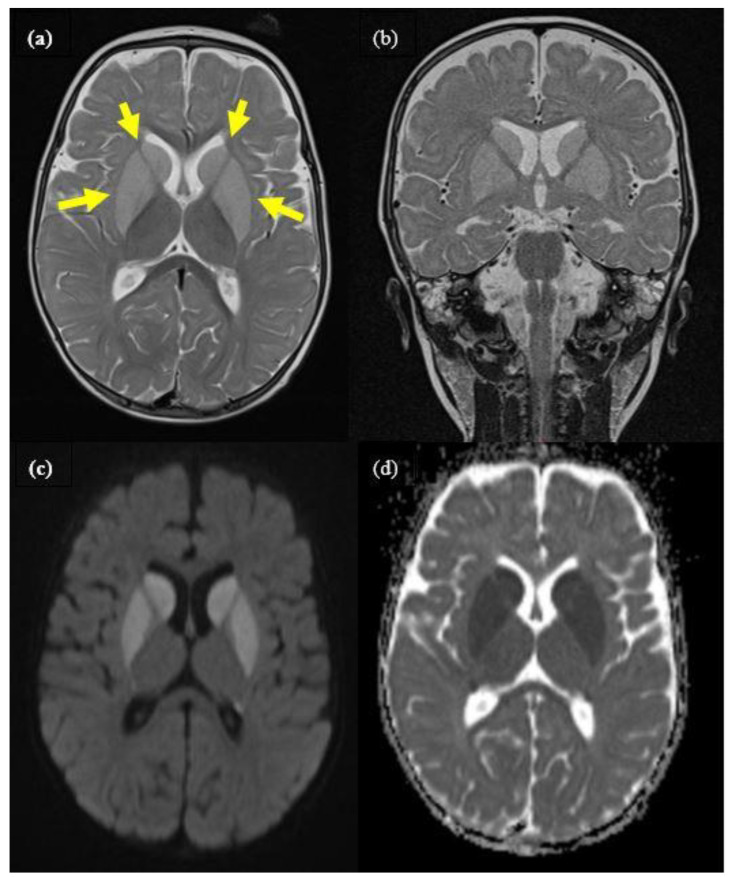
Cranial MRI of patient 1 during encephalopathic crisis at the age of 9 months, showing distinct bilateral signal alteration of caudate, putamen, and pallidum (yellow arrows). (a): axial T2, (b): coronar T2, (c): axial diffusion weighted (DWI), (d): ADC-map imaging.
